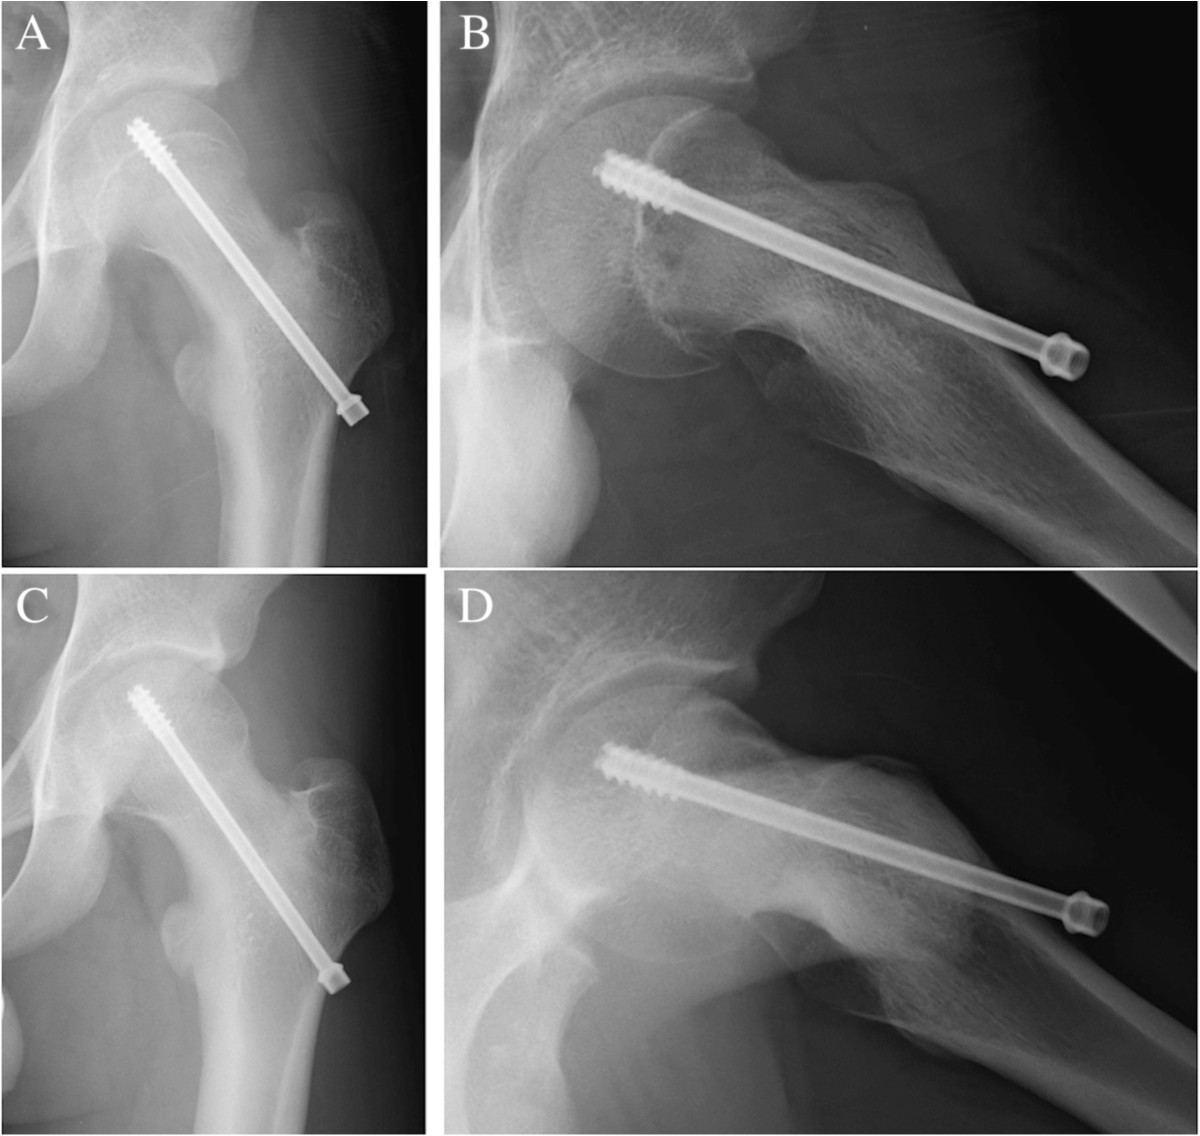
Radiographs of the left hip in a 13-year-old boy with SCFE. Anteroposterior (A) and lateral (B) radiographs immediately after in-situ fixation (ISF) for acute on chronic and stable type hips at 42 degrees of posterior tilting angle (PTA). A single screw was inserted from the lateral point. Anteroposterior (C) and lateral (D) radiographs at physeal closure. PTA was maintained without progression of the slip.
